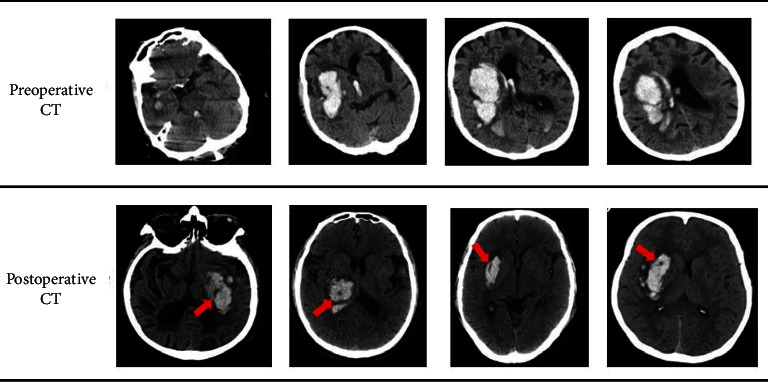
Head CT before and after surgery.
